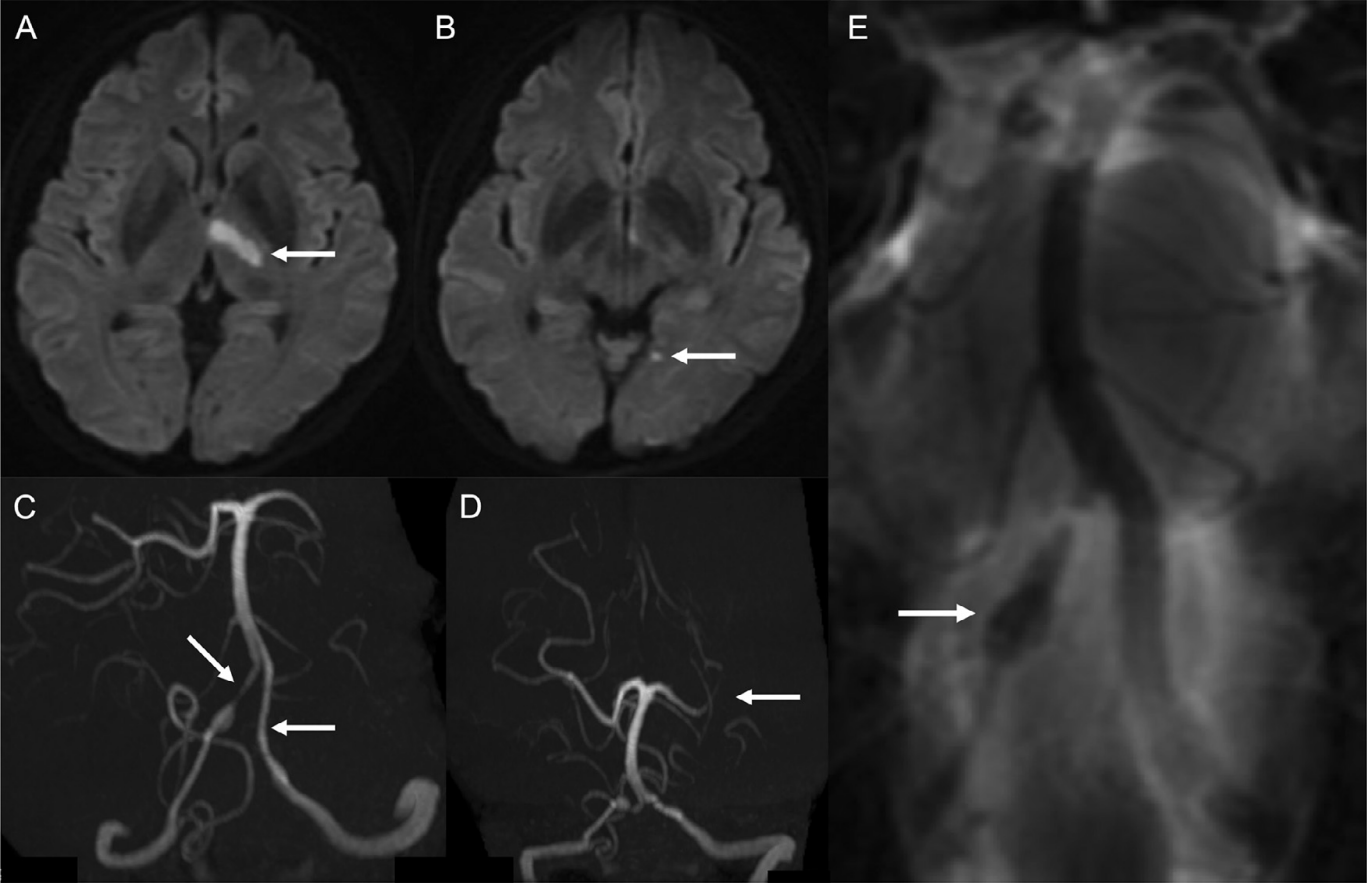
Magnetic resonance imaging on admission showing infarcts (arrows) in the left thalamus (A) and temporo-occipital lobe (B). Magnetic resonance angiography showing bilateral vertebral artery stenosis (arrows) (C) and posterior cerebral artery stenosis (arrow) (D). Basi-parallel anatomical scanning showing fusiform dilation of the right vertebral artery (arrow) (E).
